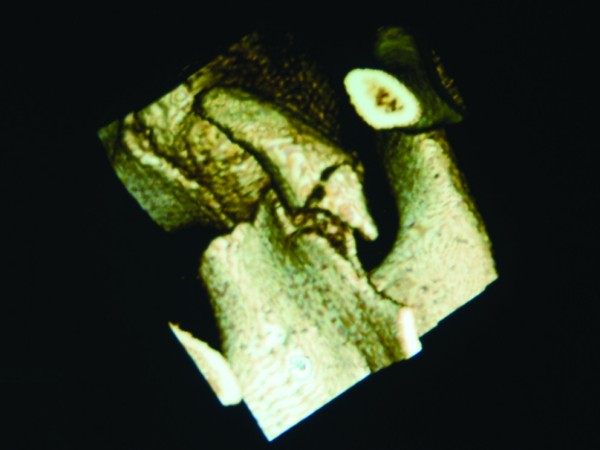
Three-dimensional reconstructions of the CT scan give a spatial view of the coracoid fracture fragment.
